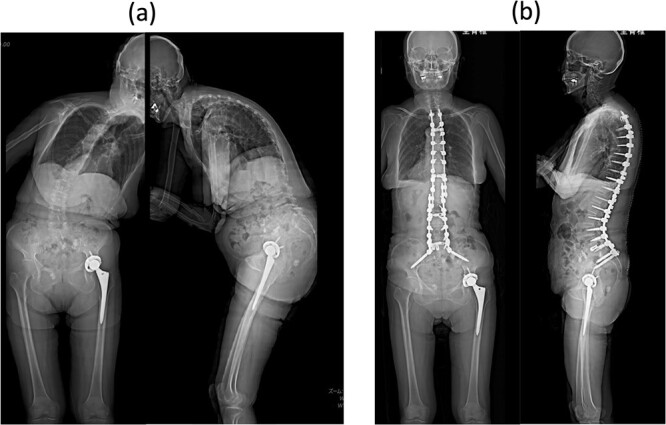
Standing whole-spine anteroposterior and lateral radiographs (a) preoperative and (b) after the first surgery.
